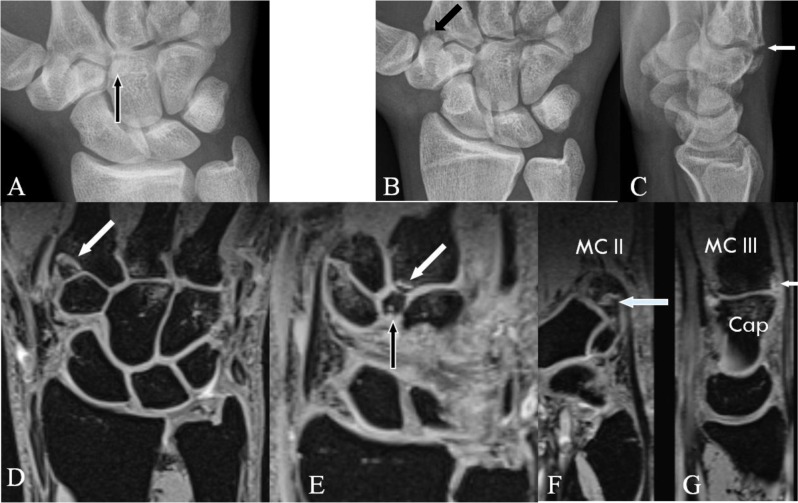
Recent fracture at the base of the second and third MC. (A–C) Plain radiography, (D–E) Coronal 3D-GRE and (F–G) Sagittal 3D-GRE. (A) Asymptomatic presence of an OS (arrow) at the CMC joint in a previous radiograph. (B–G) The patient presented three years later with elective pain at the CMC joint following trauma. (B) Fracture at the base of the second MC (arrow), (C) CB with an os styloideum (arrow), (D, F) fracture at the base of the second MC (arrow) and (E, G) the base of the third MC (only visible on MRI) (white arrow), (E) presence of an os styloideum (black arrow) and (G) rupture of the dorsal CMC ligament (arrow) with surrounding soft tissue edema. (MC = metacarpal; Cap = os capitatum).
